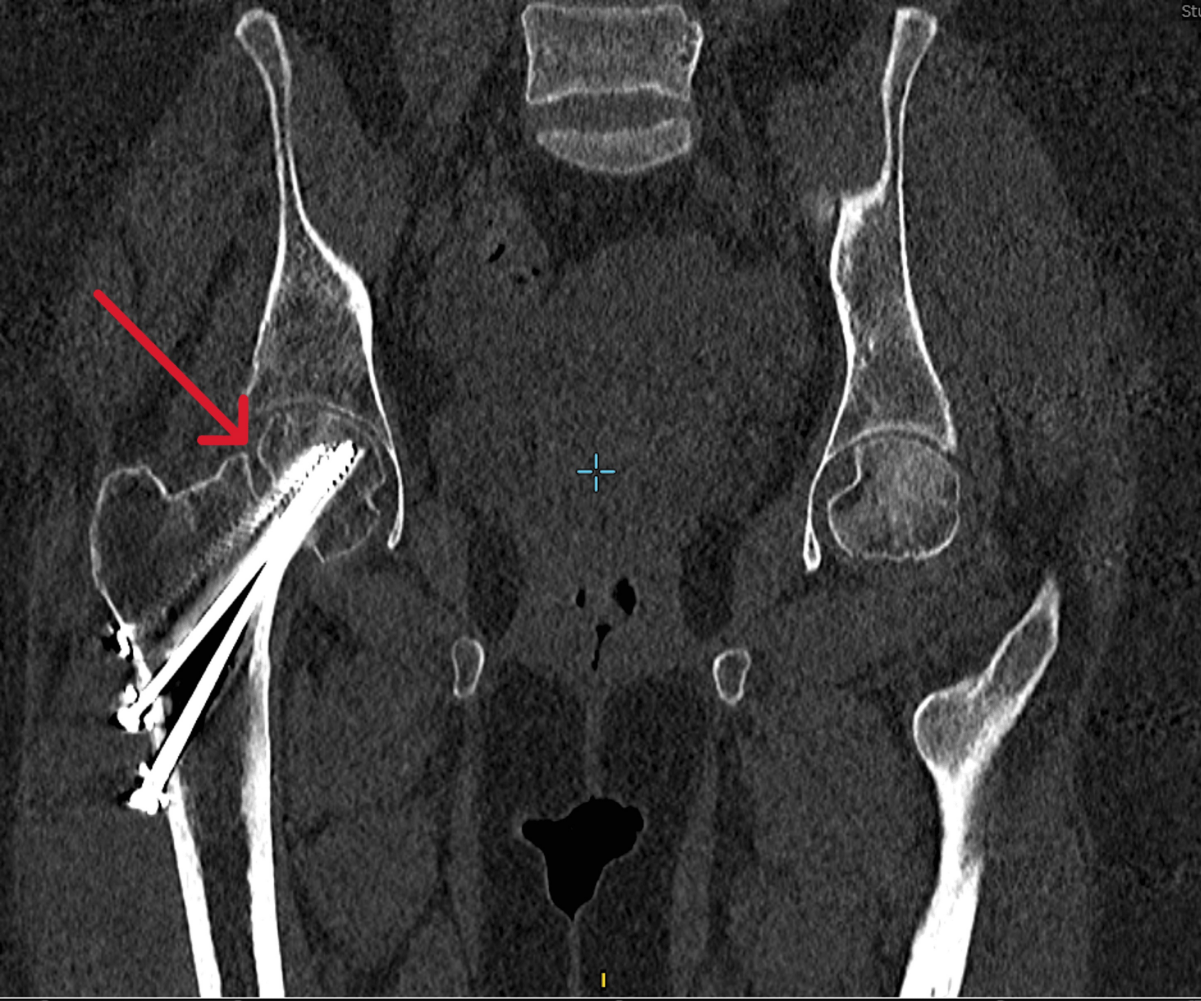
CT coronal view at one-year post-op The red arrow shows the lack of osseous bridging, along with cortical defects and well-defined fracture lines, confirming the diagnosis of non-union.
